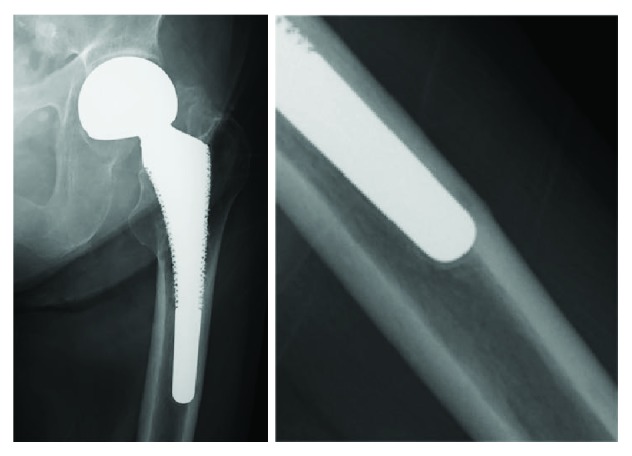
Anteroposterior pelvis radiograph taken two years prior showed that the femoral stem was in contact with the lateral side of the femur; there was no evidence of fractures and localized periosteal thickening of the lateral cortex slightly emerging in the same area.
